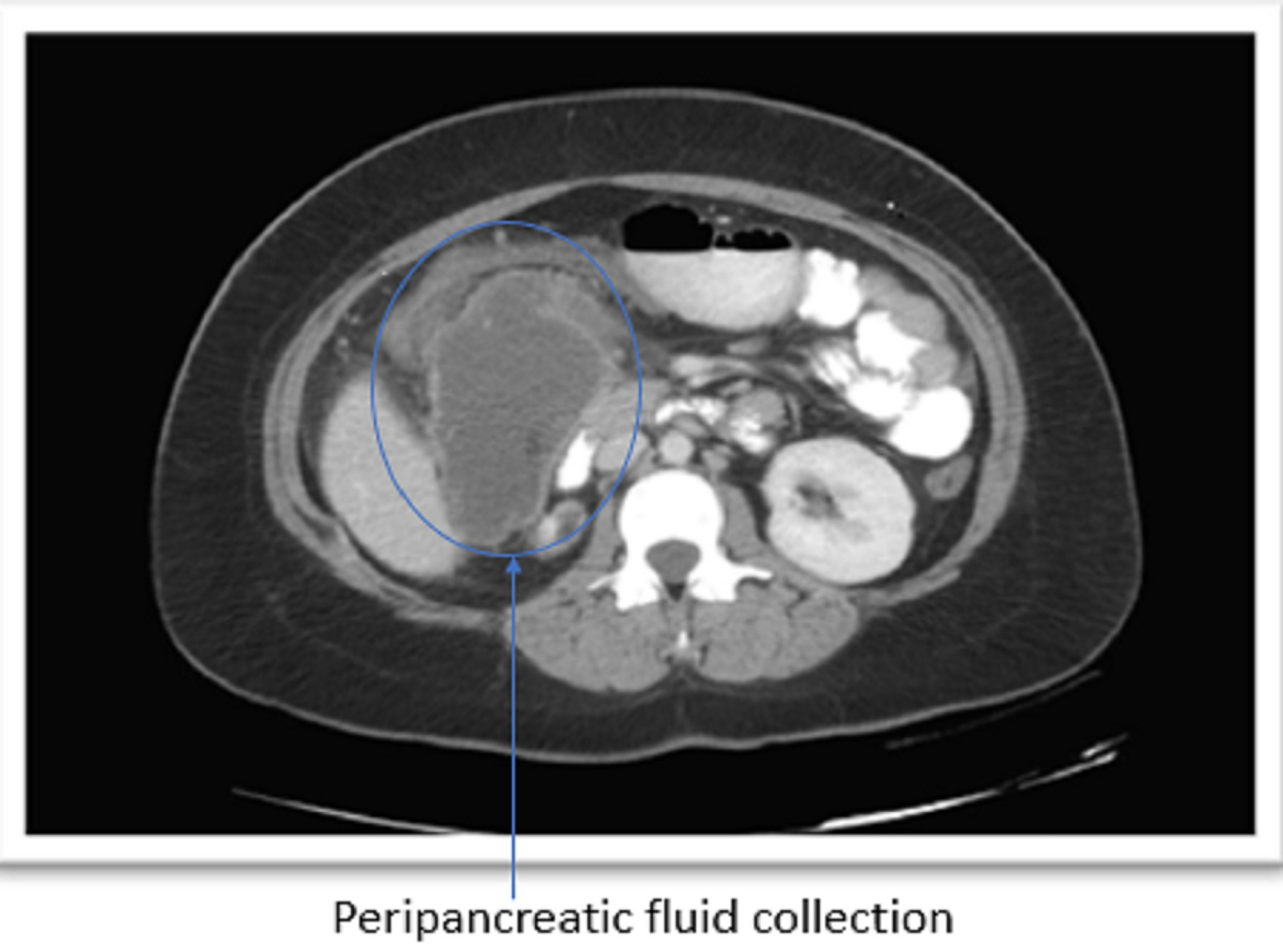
Coronal CT of the abdomen section at the level of pancreas confirming a large 7.7 x 8.8 cm rim-enhancing fluid collection.
